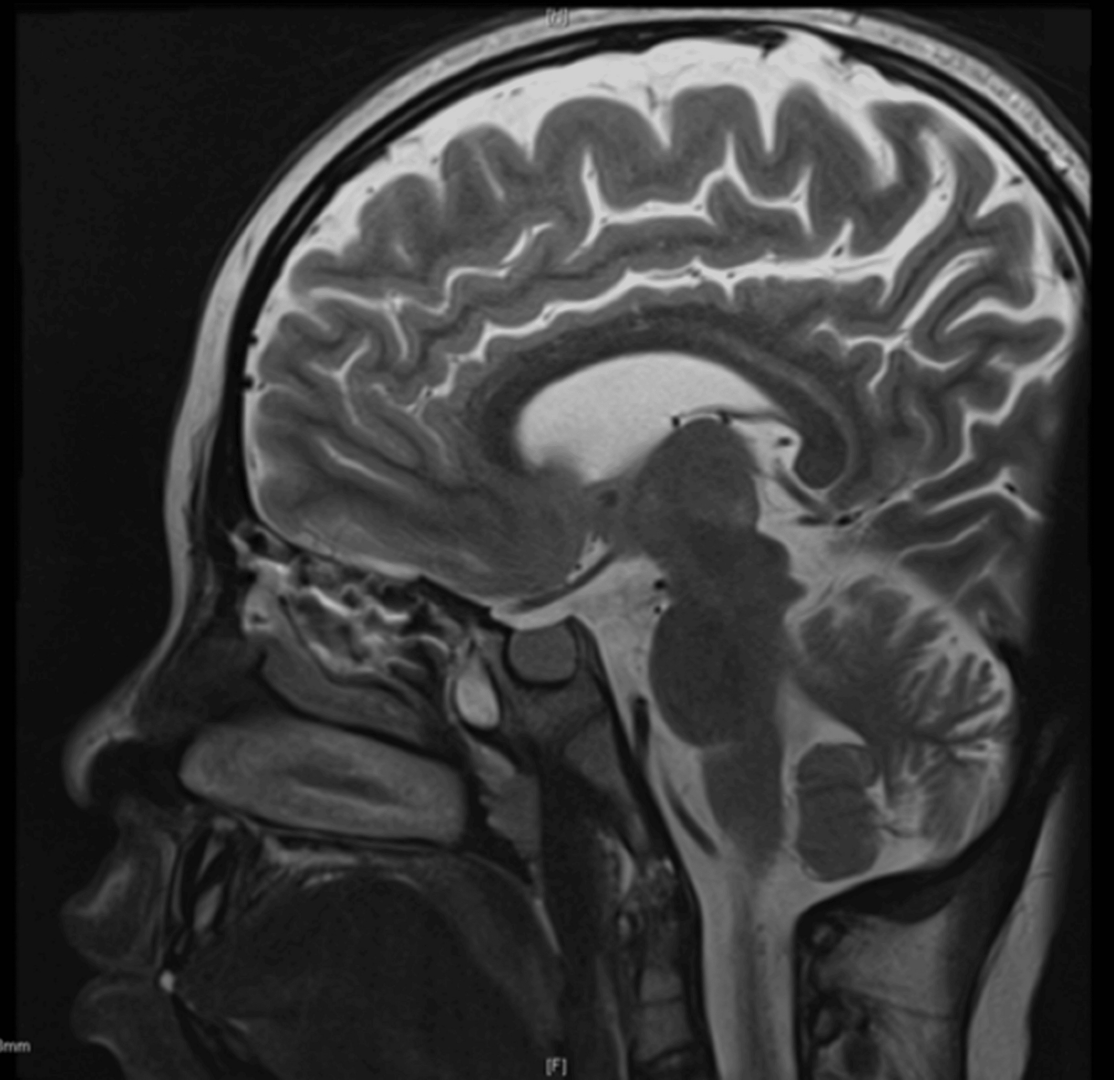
Sagittal plane of the MRI brain and pituitary of our case - showing the pituitary hyperplasia with otherwise no abnormal intensity. MRI: magnetic resonance imaging
